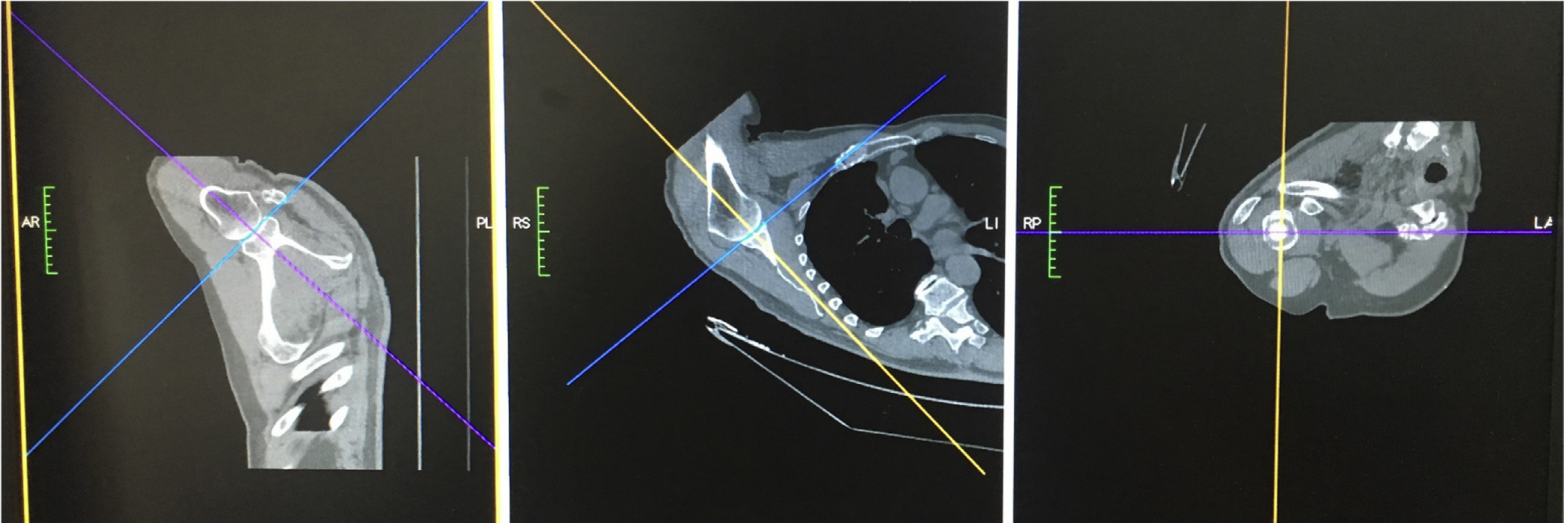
Identifying the center of the glenohumeral (GH) and the trajectories through it on 2D CT images (vertical blue, horizontal purple, reference yellow).
